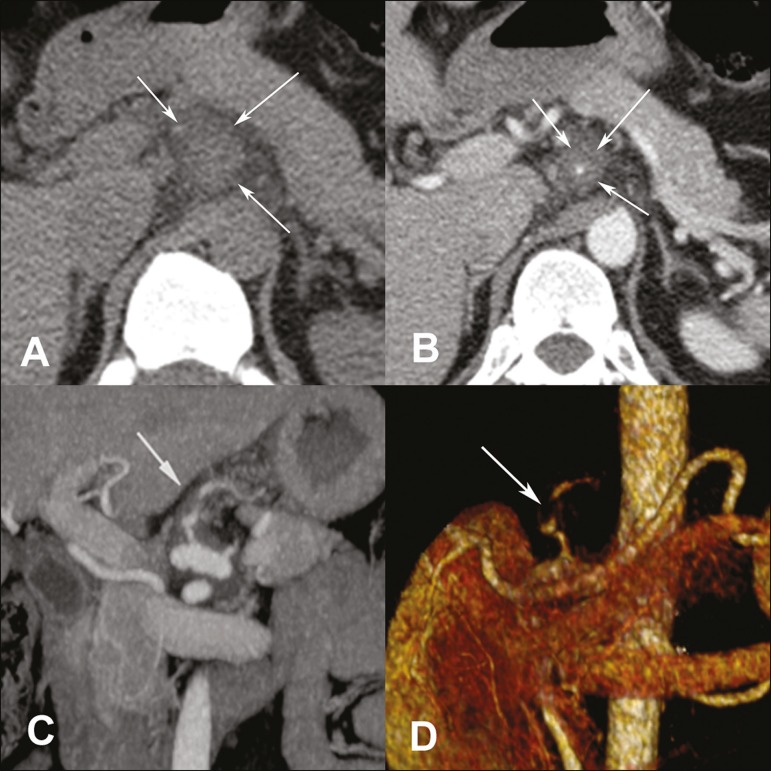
A: CT showing high attenuation (50 HU) of the tissue before
